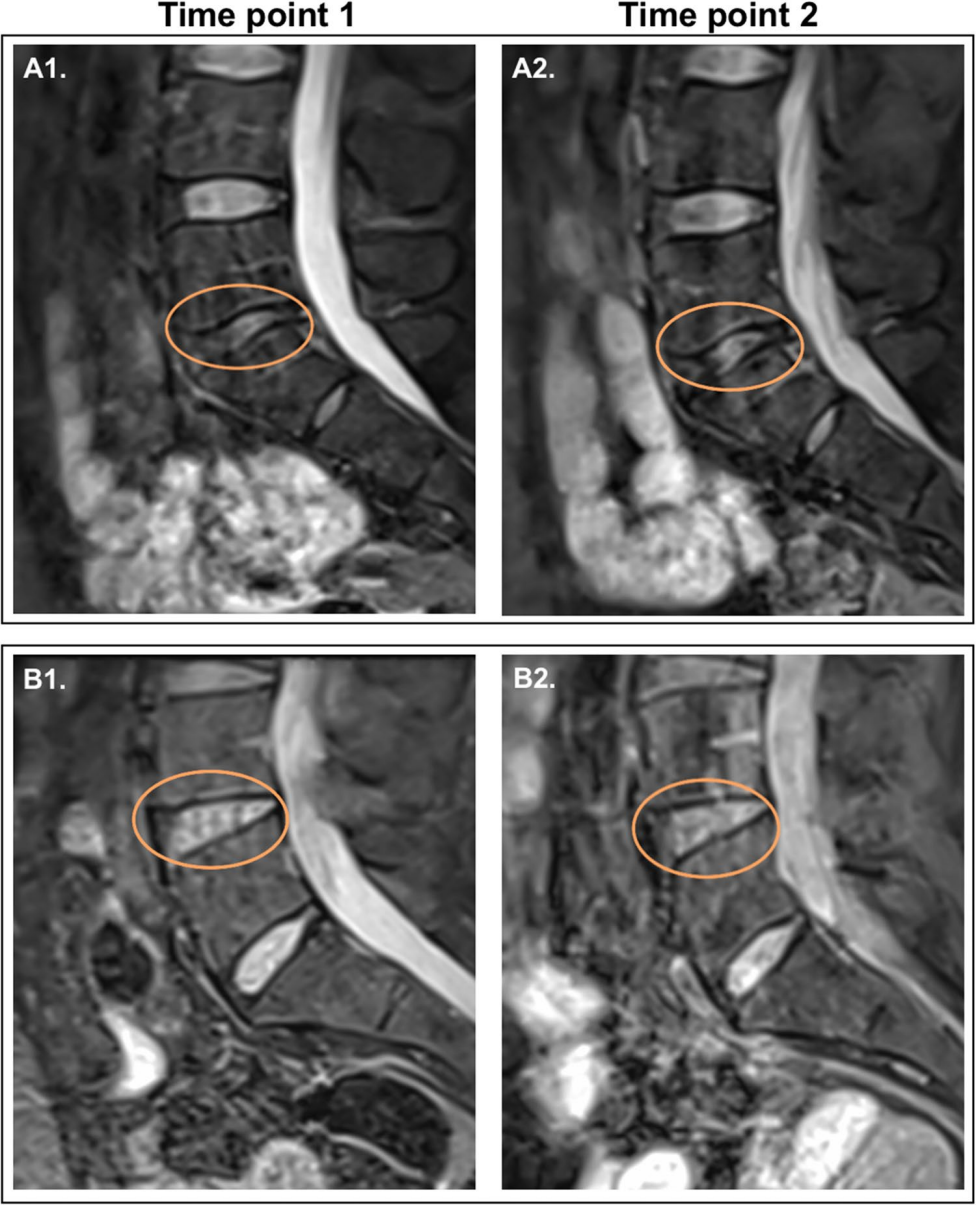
Sagittal PD FS TSE MR images of 2 asymptomatic participants: A moderate disc degeneration (grade 3) at L5–S1 at time point 1 (A1) and no worsening at time point 2 (A2) in a 45-year-old man; B mild disc degeneration (grade 2) at L4–L5 at time point 1 (B1) and no worsening at time point 2 (B2) in a 58-year-old woman
